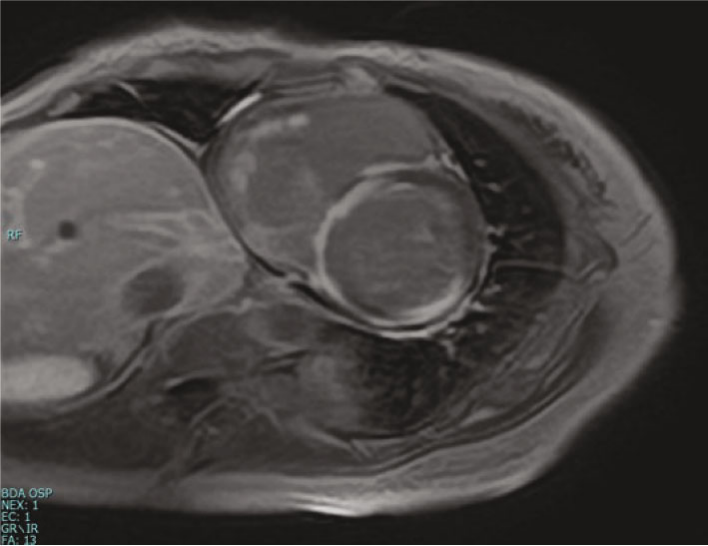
Cardiac magnetic resonance imaging. The image reveals significant late gadolinium enhancement in the basal septal to the inferior wall of the LV and the RV septal wall.
